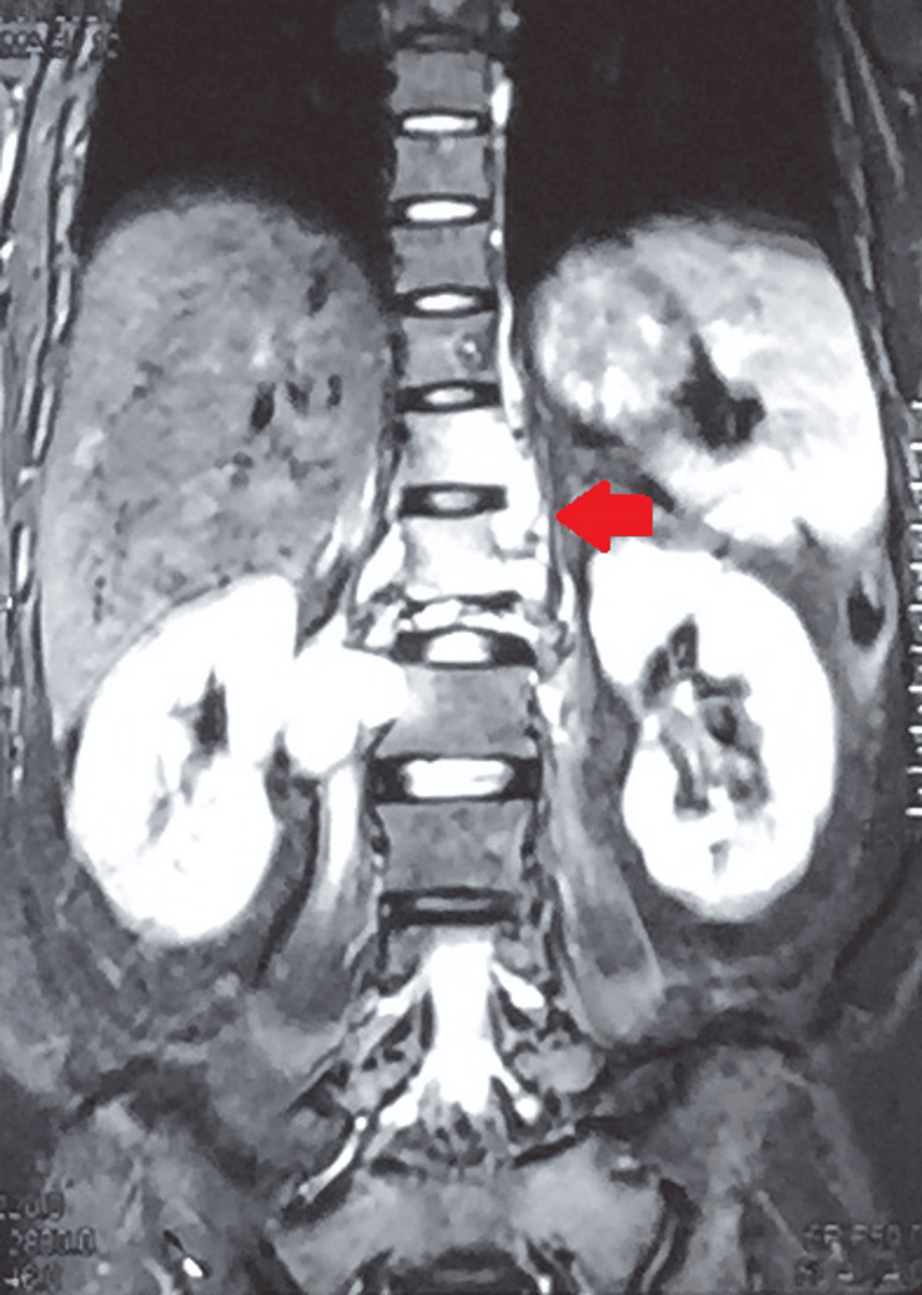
MRI of the dorso-lumbar spine with multiple tiny pre- and paravertebral collections
